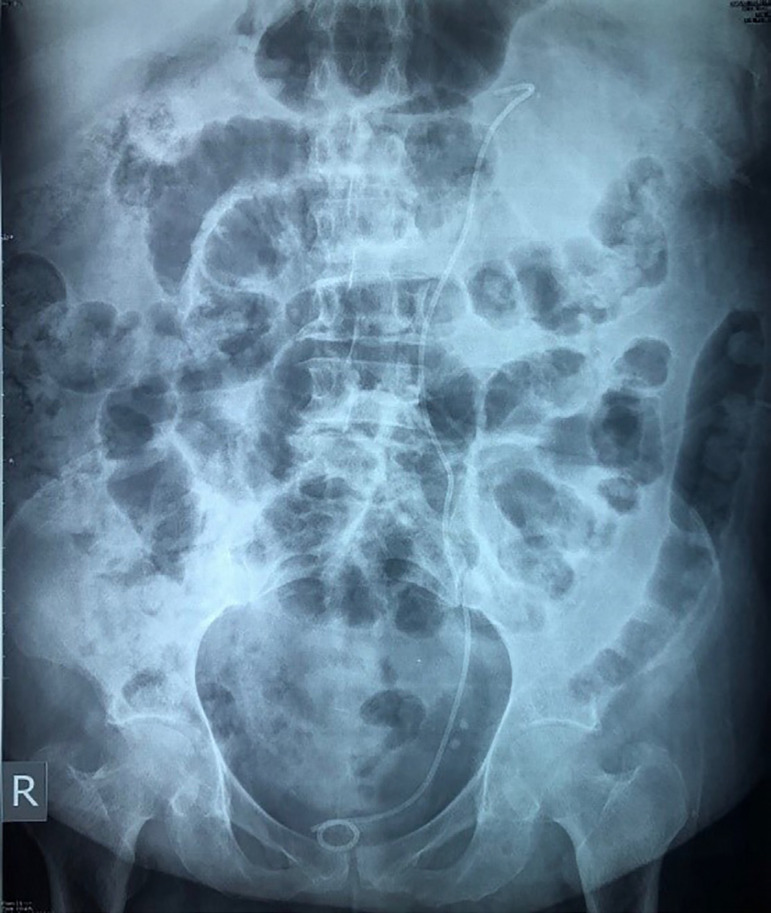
X-ray KUB showing the left JJ in place, as well as the diffuse colonic distention corresponding to ileus post colonoscopy.
